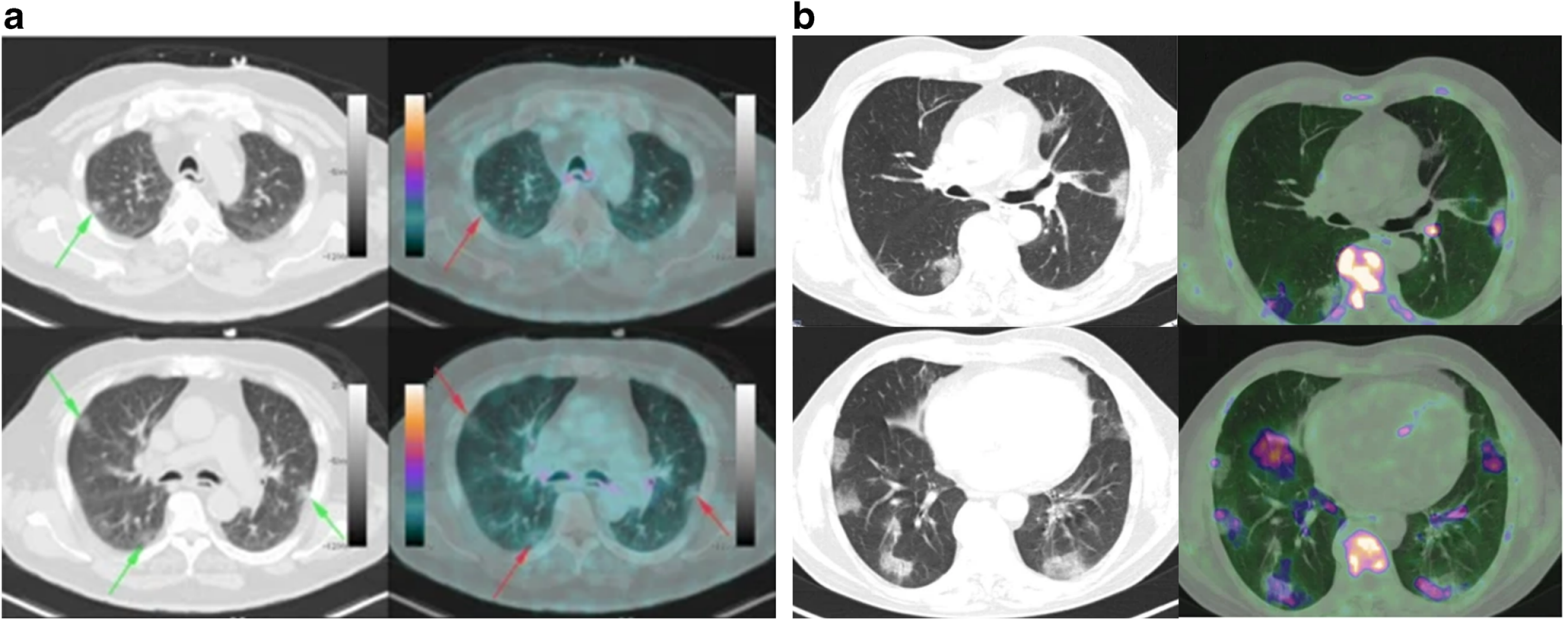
Examples of PET/CT images of COVID-19 patients using ^68^Ga-PSMA-11 and ^18^F-choline. a
